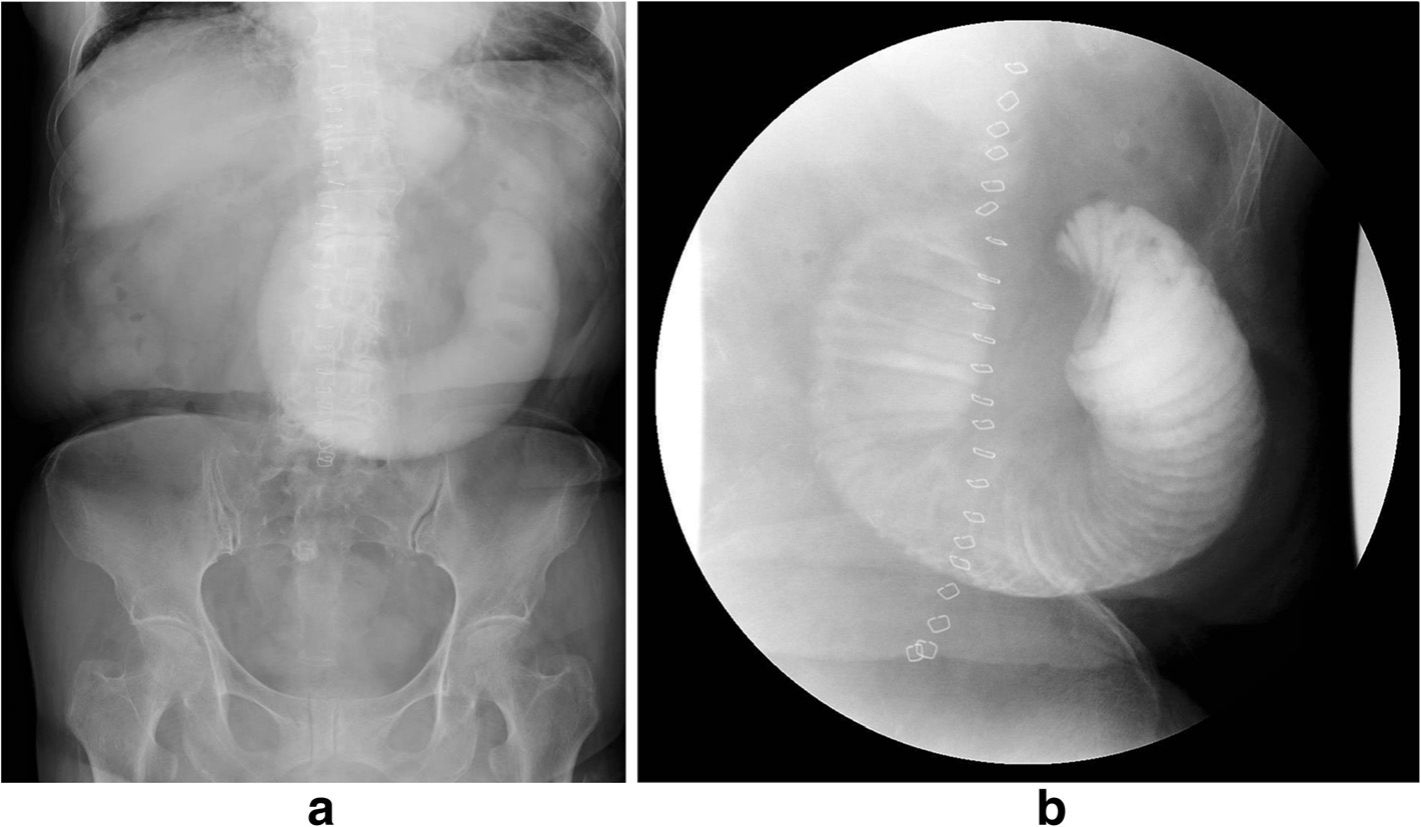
Abdominal X-ray picture and upper gastrointestinal series findings. Abdominal X-ray picture (a) and upper gastrointestinal series (b) showed decreased peristalsis and distension of the elevated jejunum without visible passage to the anal intestine
